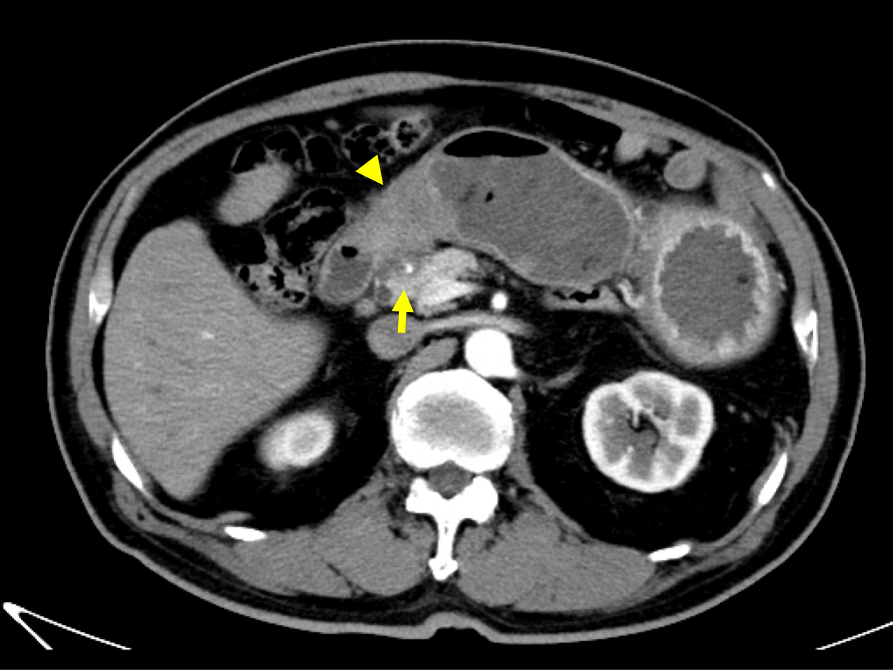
Four months later, abdominal CT scan showing a more thickened wall than that observed in previous CT scans (arrow heads). The lesion involved the surrounding tissue of the gastroduodenal artery, suggesting invasion (arrow)
